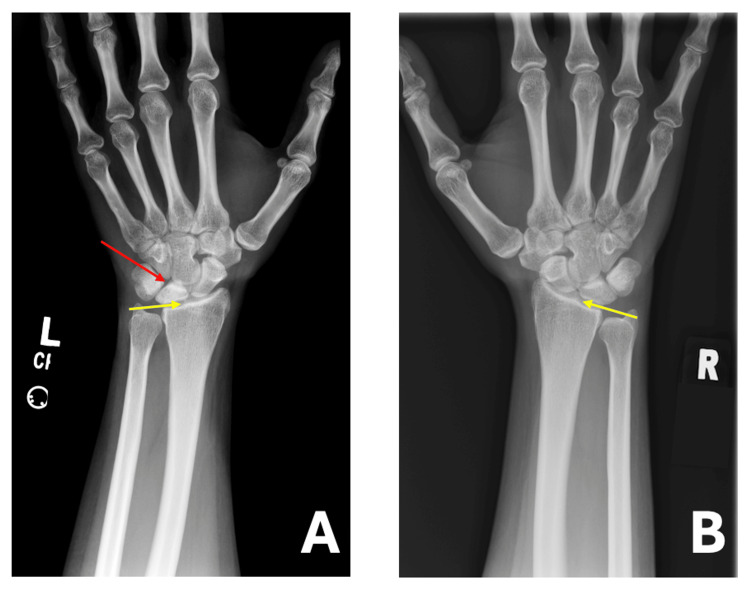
(A, B) Bilateral PA wrist radiographs. (A) Left wrist: The yellow arrow indicates negative ulnar variance, while the red arrow highlights lunate density. (B) Right wrist: The yellow arrow represents negative ulnar variance, with no evidence of lunate alteration. PA: posteroanterior.
